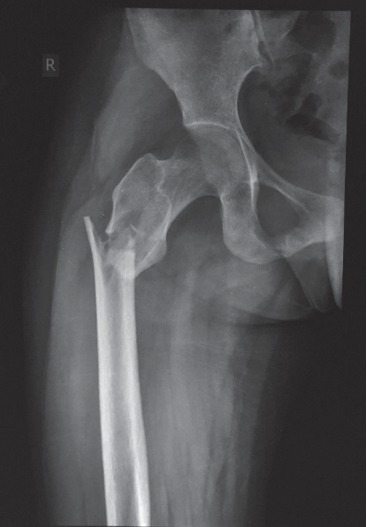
X-ray showing the right subtrochanteric fracture.
